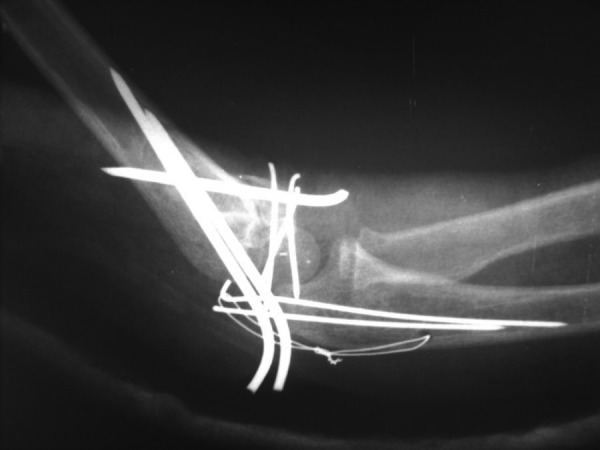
Control X-Ray After 3 Weeks Postoperative
